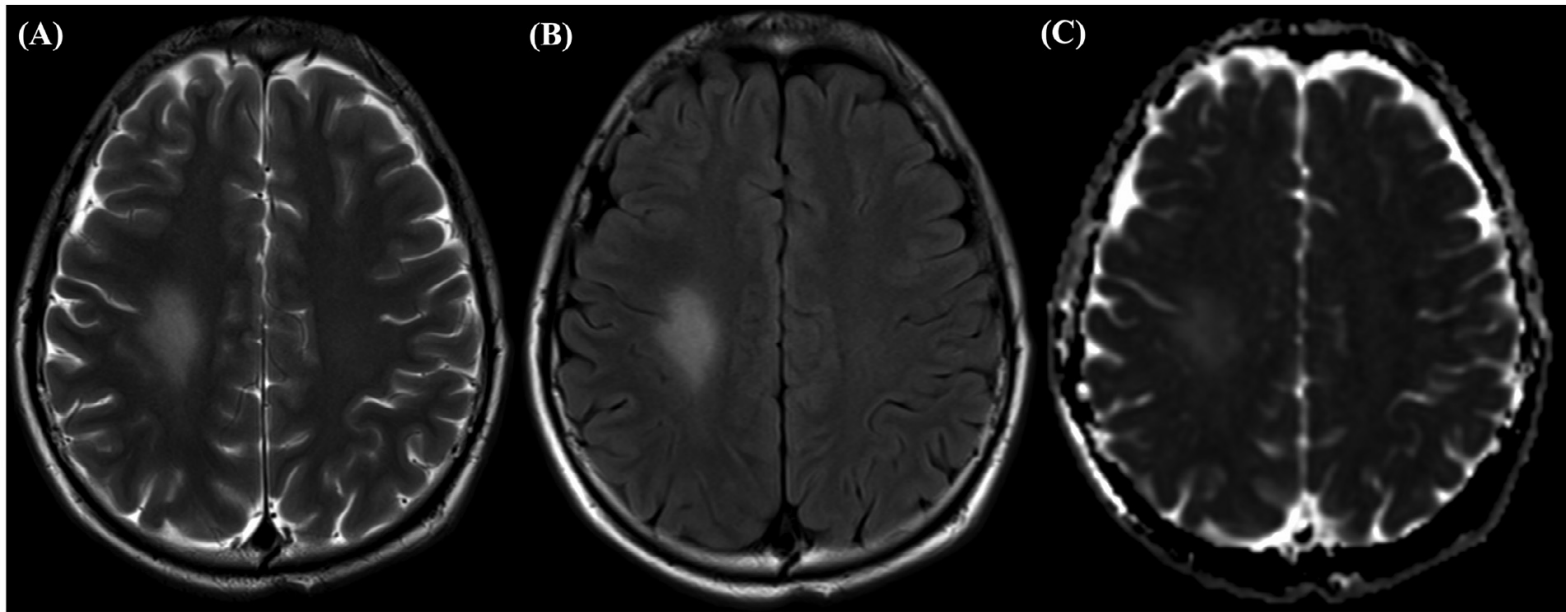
MRI findings taken one month after presentation, revealing asymmetric well-defined hyperintensity in the right centrum semiovale without corresponding diffusion restriction on (A) T2-weighted image, (B) FLAIR, (C) DWI.
